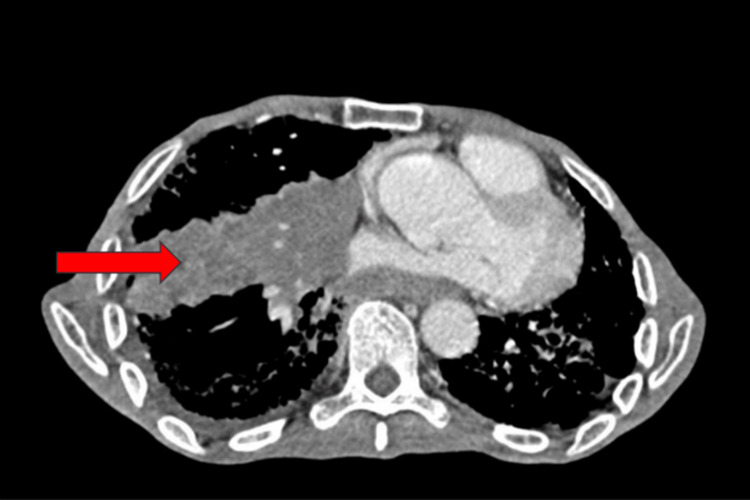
Thoracic CT scan with mediastinal window showing the presence of a right mediastinal-pulmonary lesion. Red arrow: Mediastinal-pulmonary lesion in the middle lobe, poorly defined, containing calcifications, measuring approximately 126 x 82 mm, extending over 200 mm.
